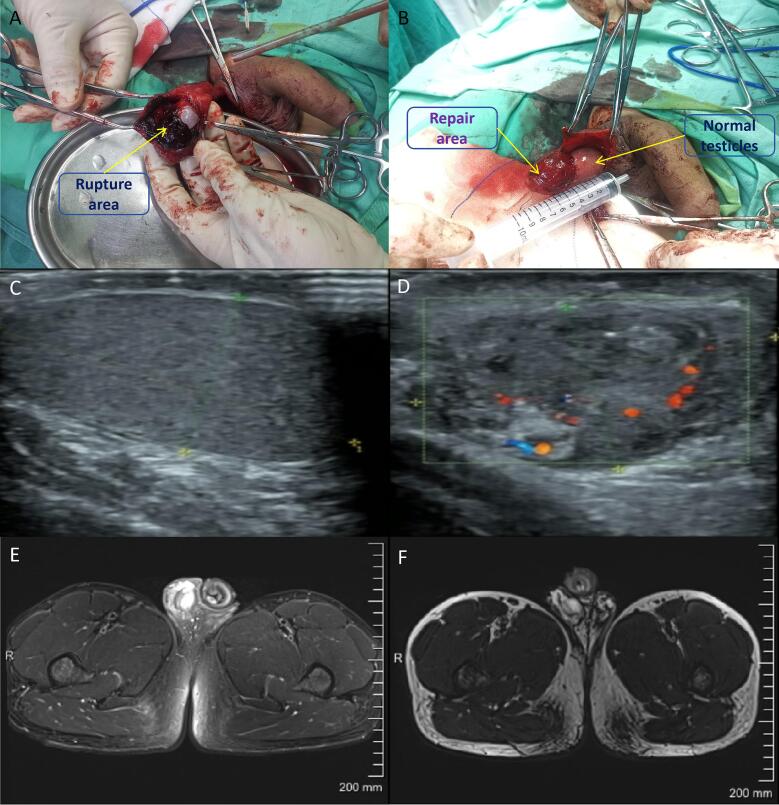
(A) Intraoperative tunica albuginea rupture was observed, with a rupture of approximately 1 cm. (B) Repair of tunica albuginea defect with tunica vaginalis, approximately 3 cm in length. (C) Six months after surgery, ultrasound examination revealed testicular morphology. (D) Six months after surgery, ultrasound showed good testicular blood supply. (E) Three months after surgery, MRI showed good testicular recovery. (F) Six months after surgery, MRI showed a reduction in testicular volume.
